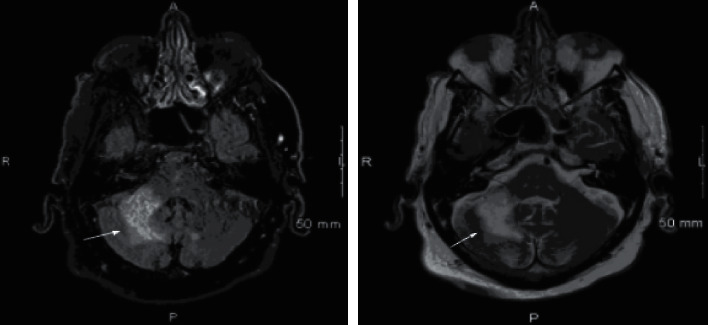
MRI showing interval increase in size of T2 and FLAIR hyperintense infiltrative signal intensity in the right middle cerebellar peduncle and right cerebellar hemisphere as well as a small new focus of FLAIR hyperintense signal intensity in the left cerebellar hemisphere parasagittal to midline.
